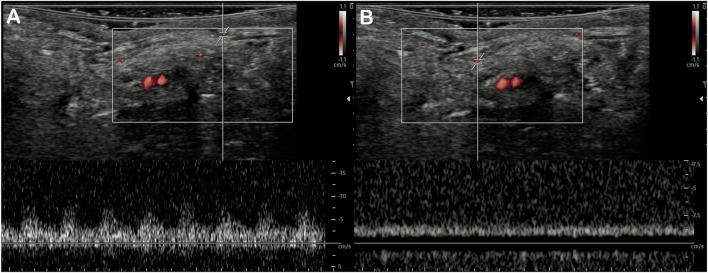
Detection of superficial microvessels by MV-Flow. The use of pulsed Doppler with a positioned recording area at the MV-Flow detection site allows differentiating small arteries with the pulsed flow (A) from small veins with the continuous flow (B) in the same field of view.
